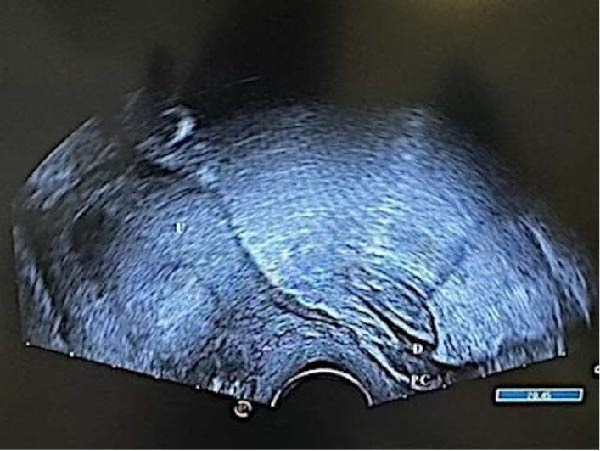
Douglas view on vaginal ultrasonography during Pezzer catheter removal.
